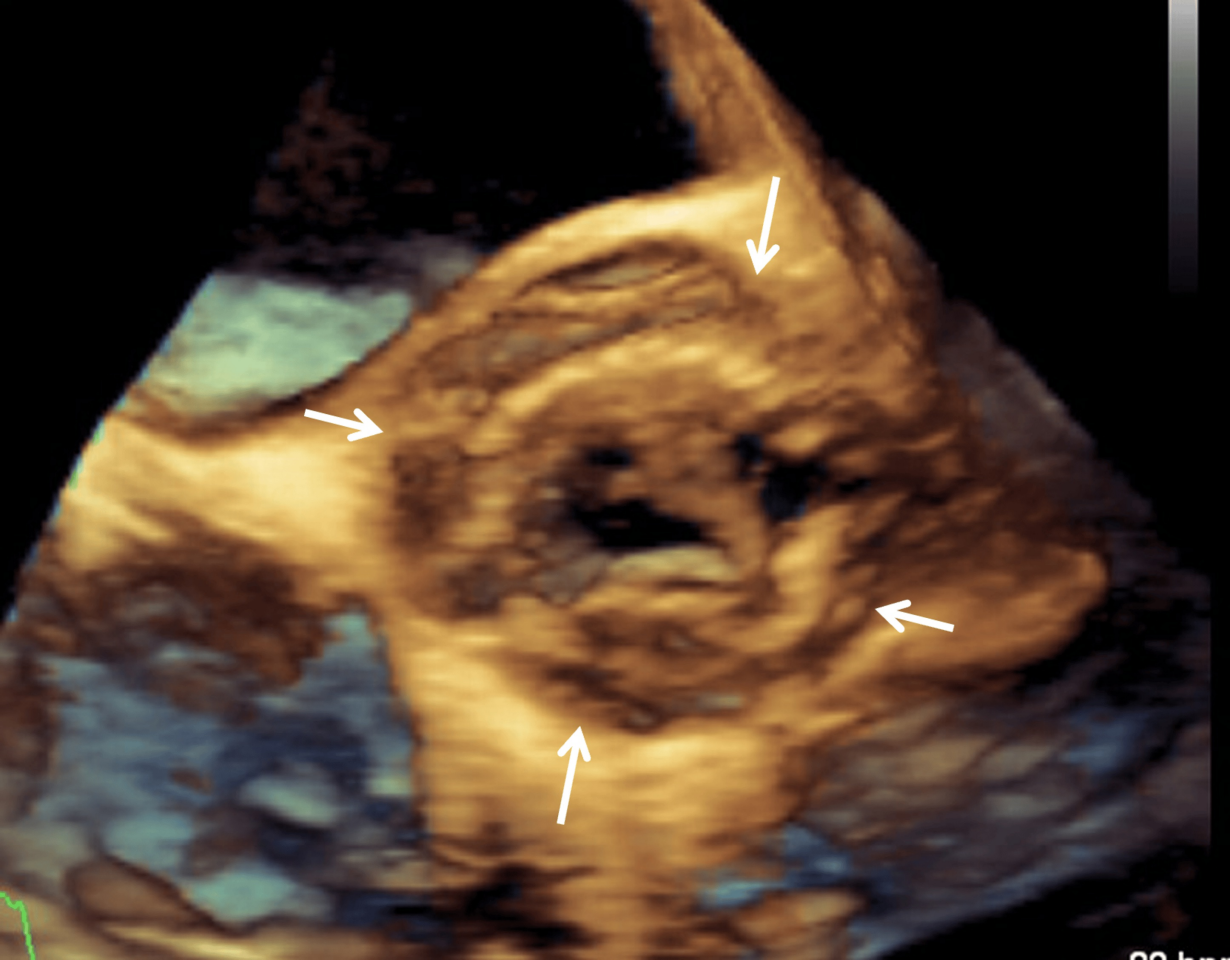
Transesophageal echocardiography shows the aortic bioprosthesis surrounded by a periannular abscess on 3D images (arrows).
